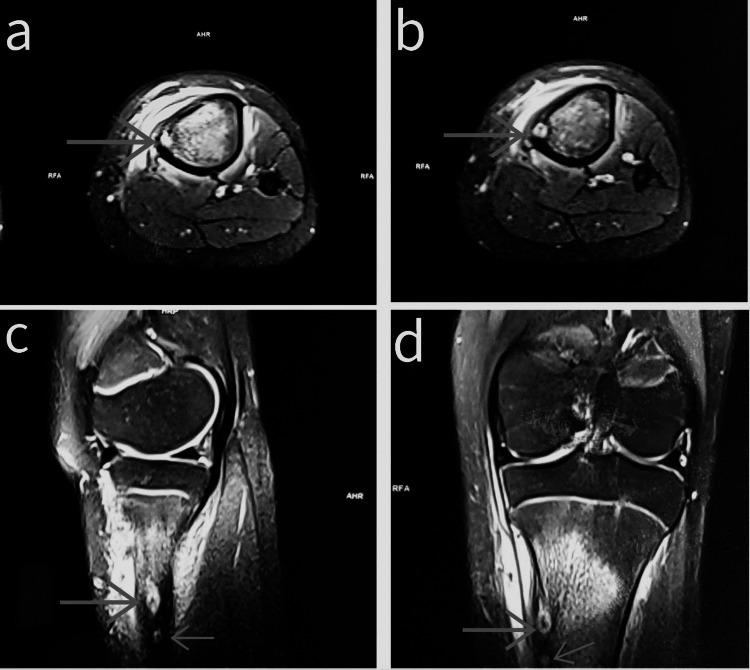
Magnetic resonance imaging short tau inversion recovery (MRI STIR) sequences axial (a,b), sagittal (c), and coronal (d) images showing an osteoid osteoma with two nidi Two small, eccentric, hypointense lesions on STIR sequences with peripheral hyperintense rims within the cortex (arrows showing two nidi).
